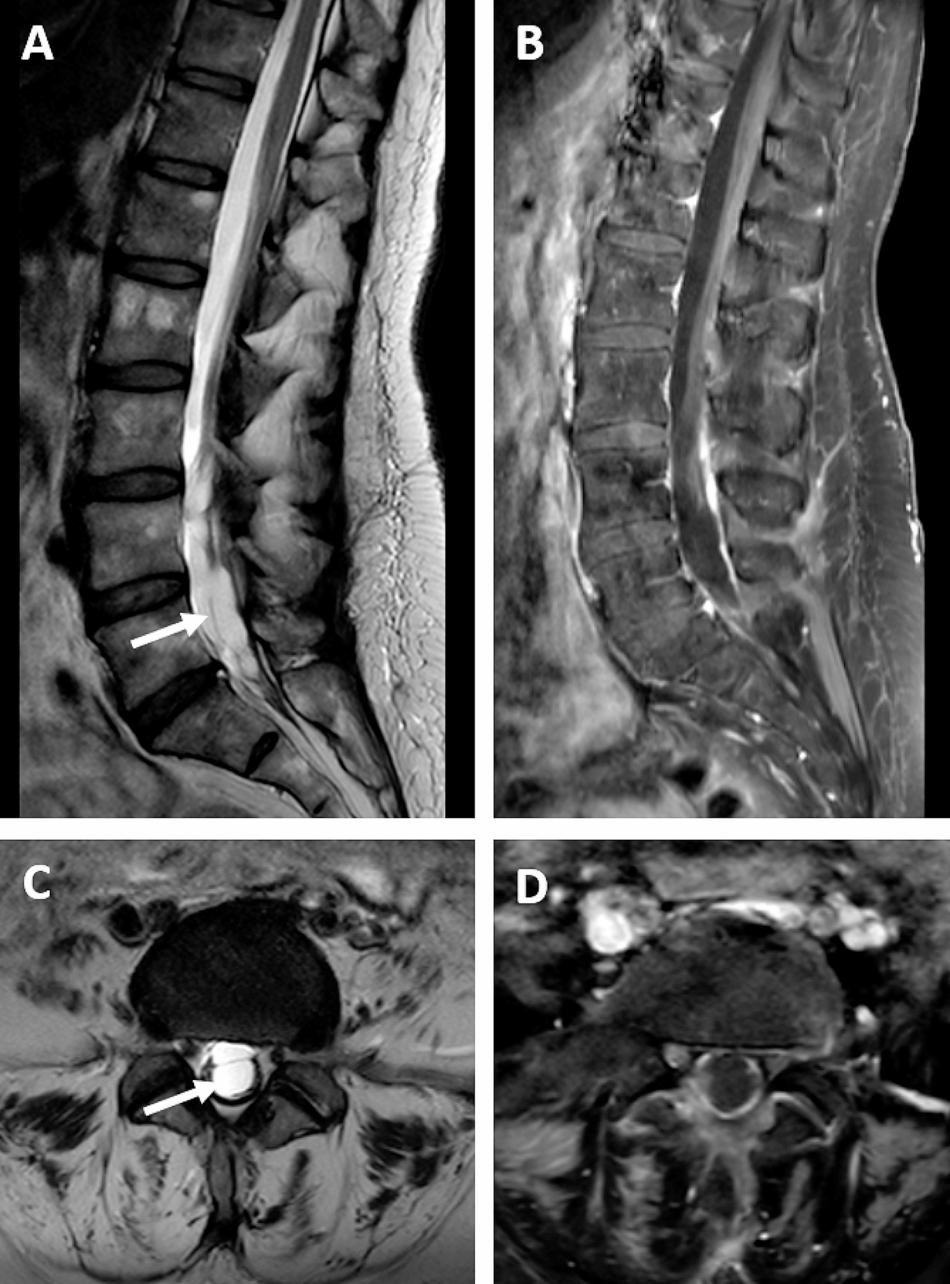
MRI of the lumbosacral spine performed on a 3T scanner, T2-weighted (A, C) and T1-weighted images after contrast administration (B, D). MR examination revealed the distorted nerve roots, adherent to the thecal sac, creating the “empty thecal sac” sign (arrow) typical for type II arachnoiditis
